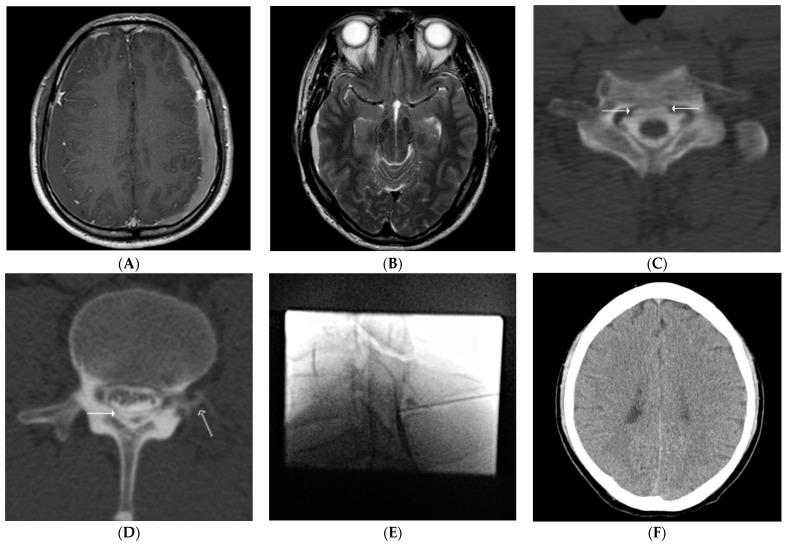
(A–F). Findings for a representative case involving a 41-year-old man with nontraumatic intracranial subdural hematoma (SDH). The patient presented with a chief complaint of headache not related to a specific posture. (A). Contrast-enhanced, fat-suppressed T1 weighted image shows bilateral nontraumatic SDH with pachymeningeal thickening and enhancement. (B). T2 weighted image at the initial presentation shows cisternal obliteration. (C,D). Computed tomography (CT) myelography performed to evaluate possible spontaneous intracranial hypotension shows cerebrospinal fluid leaks at the level of the C6/7 ventral epidural space (solid arrows, (C)), the L2/3 dorsal epidural space (a solid arrow, (D)), and the left extraforaminal space (a hollow arrow, (D)). (E). Subsequent epidural blood patch is performed at the C6/7 and L2/3 (not shown) levels. (F). Follow-up brain CT performed after 3 months shows no evidence of SDH.
